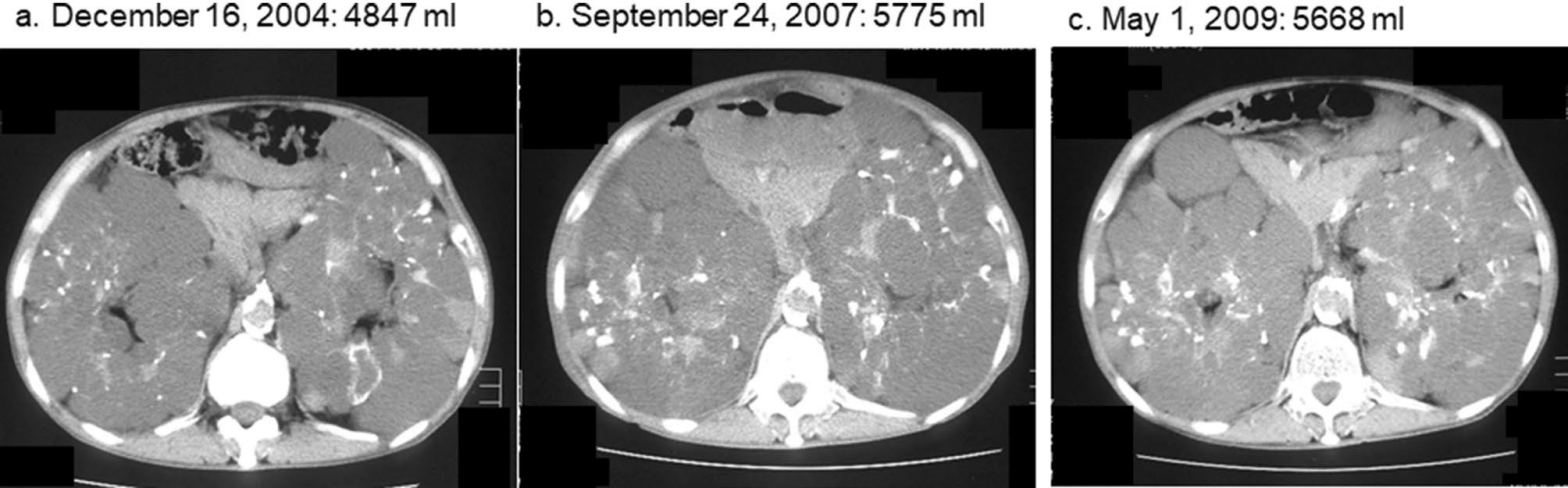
A representative case, abdominal computed tomography images (Case 3). Total kidney volume continued to increase after initiation of hemodialysis [(a) 4847 ml, December 16, 2004, (b) 5775 ml, September, 24 2007]. Cinacalcet administration was started on April 9, 2008. Total kidney volume did not increase during the cinacalcet treatment period [(c) 5668 ml, May 1, 2009].
